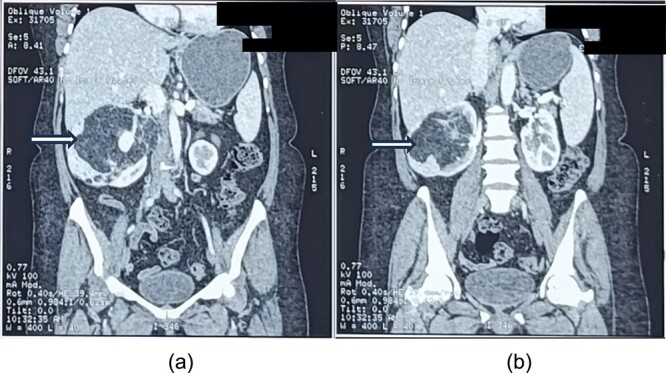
a and b showing abdominal CT scan cuts, showing mixed density mass arising from the right kidney (white arrows).
